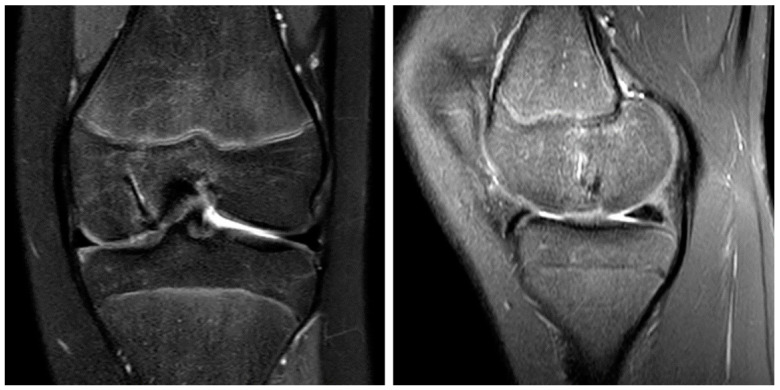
The MRI 6 months after surgery shows the OCD fragment in place and the remodeling process of the magnesium screws in the coronar (left) and sagittal plane (right).
